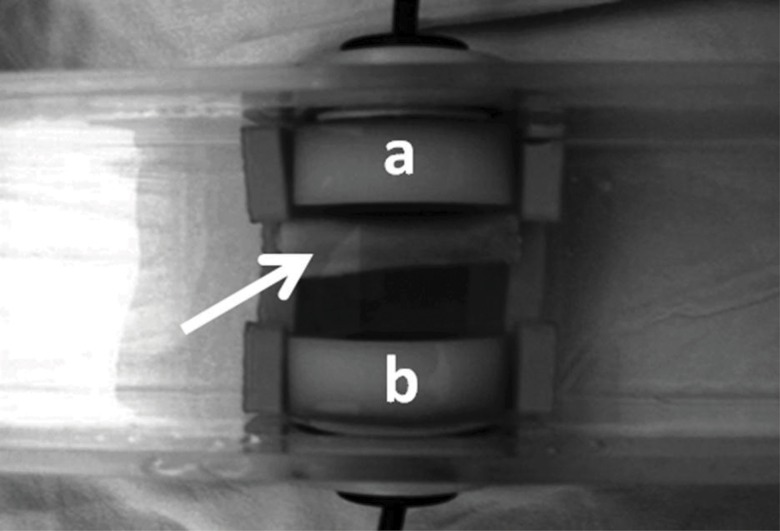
The diaphyseal segment inside the acoustic tank (arrow) positioned between the transmitting (a) and receiving (b) ultrasound transducers.
